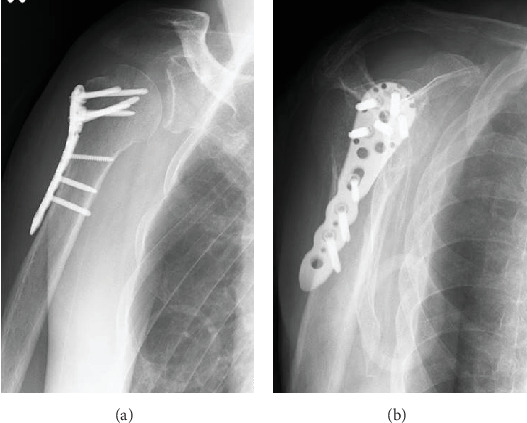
Plain radiographic images on postoperative Day 1. (a) AP view, (b) Y view.
